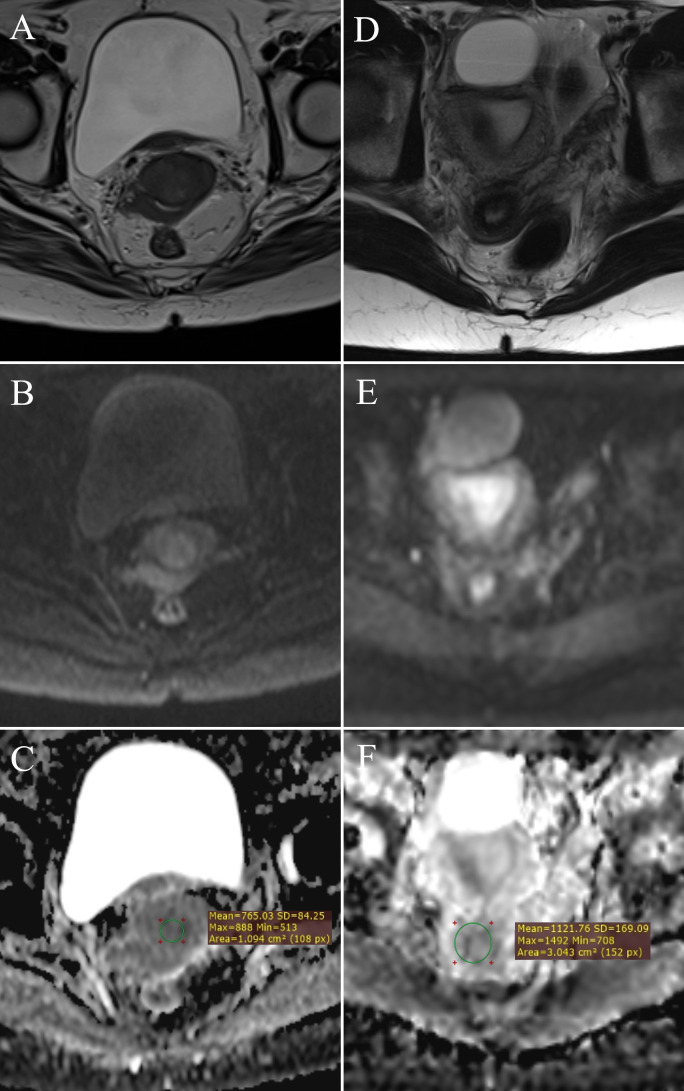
ADC value measurement: FIGO IIB stage cervical cancer – (A) (T2W), (B) (DWI), (C) (ADC map with ROI); normal cervix – (D) (T2W), (E) (DWI), (F) (ADC map with ROI).
